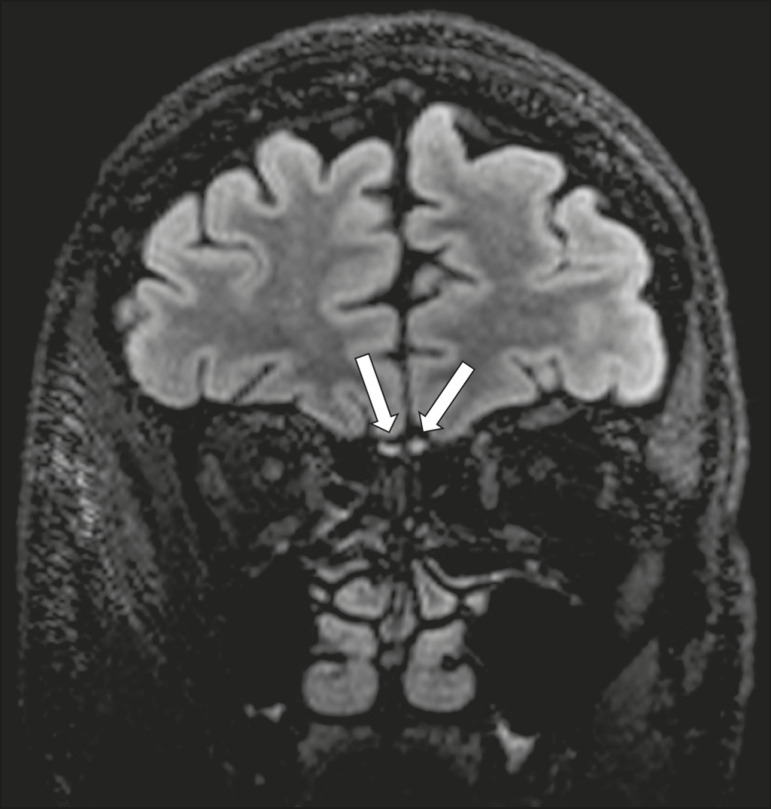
Olfactory bulb trauma. A 35-year-old male with anosmia after cranial trauma. Coronal fluid-attenuated inversion recovery MRI sequence showing a hyperintense signal in the olfactory bulbs (arrows).
